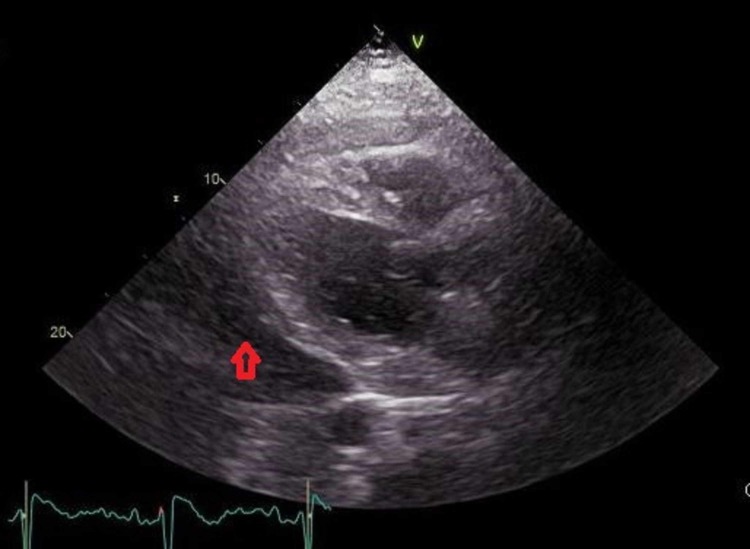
Transthoracic echocardiogram showed a large circumferential pericardial effusion (red arrow)
